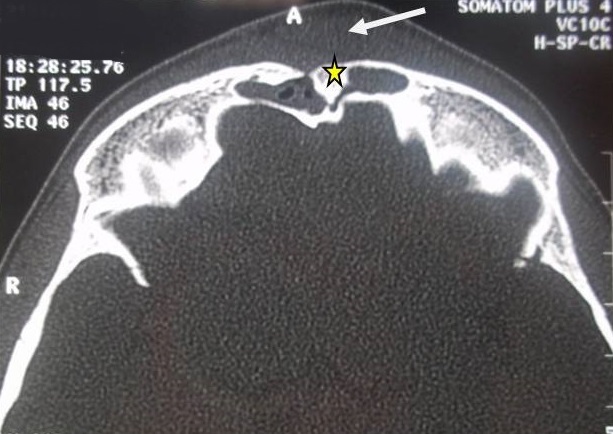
Brain CT in axial section, bone window showing a post-traumatic bone embedment of the external table of frontal sinus (star) filling and marked thickening of the soft opposite parts (arrow)
